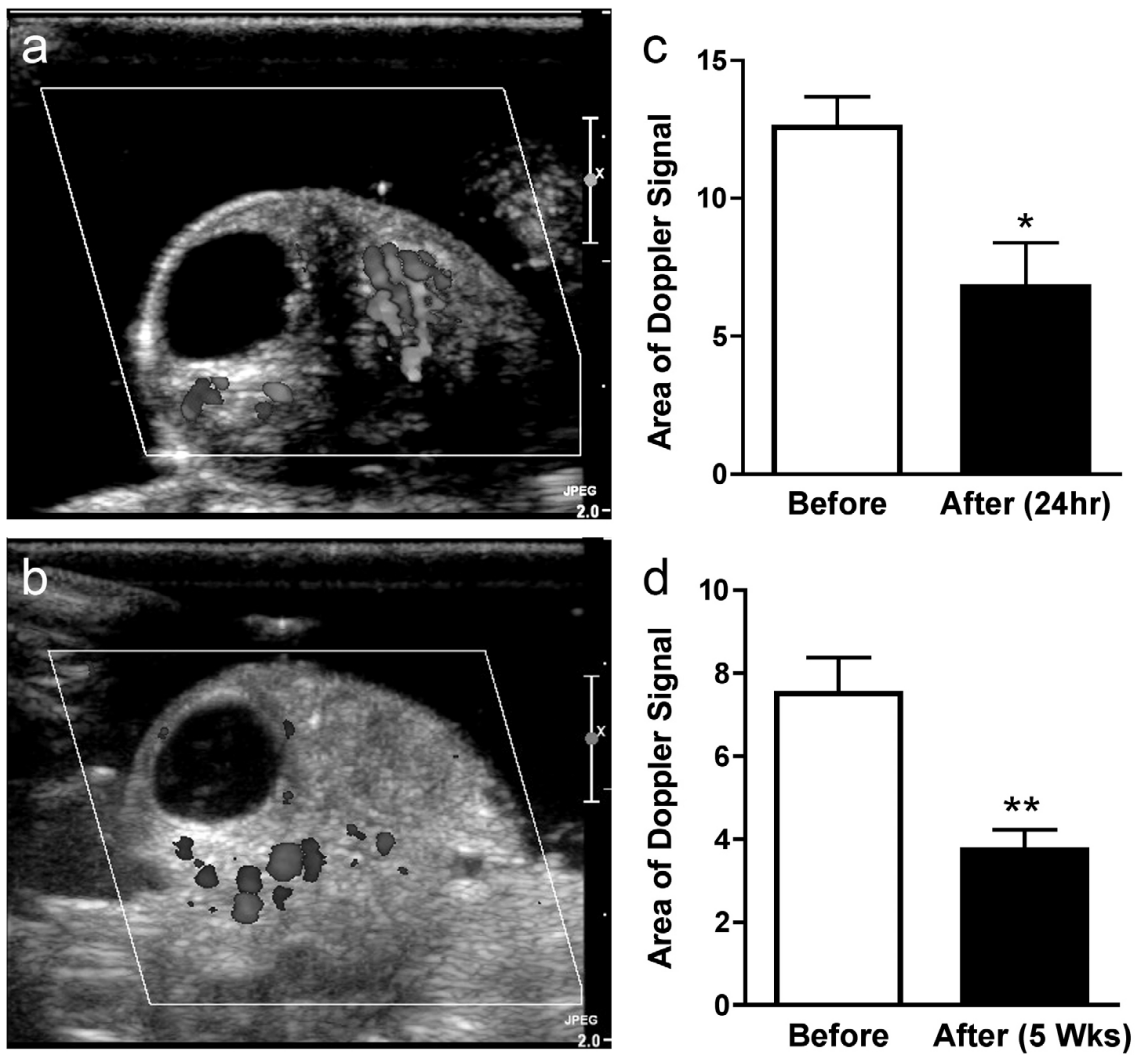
The effect of diathermy on Colour Doppler signal in the ovarian stroma. Representative ovarian scans with fixed threshold Colour Doppler signals visible before (a) and 24 hours after ovarian diathermy (b). Area of fixed threshold Colour Doppler signal in the ovary before and 24 hours (c) and 5 weeks (d) after diathermy. P*<0.05; *P*<0.01.
